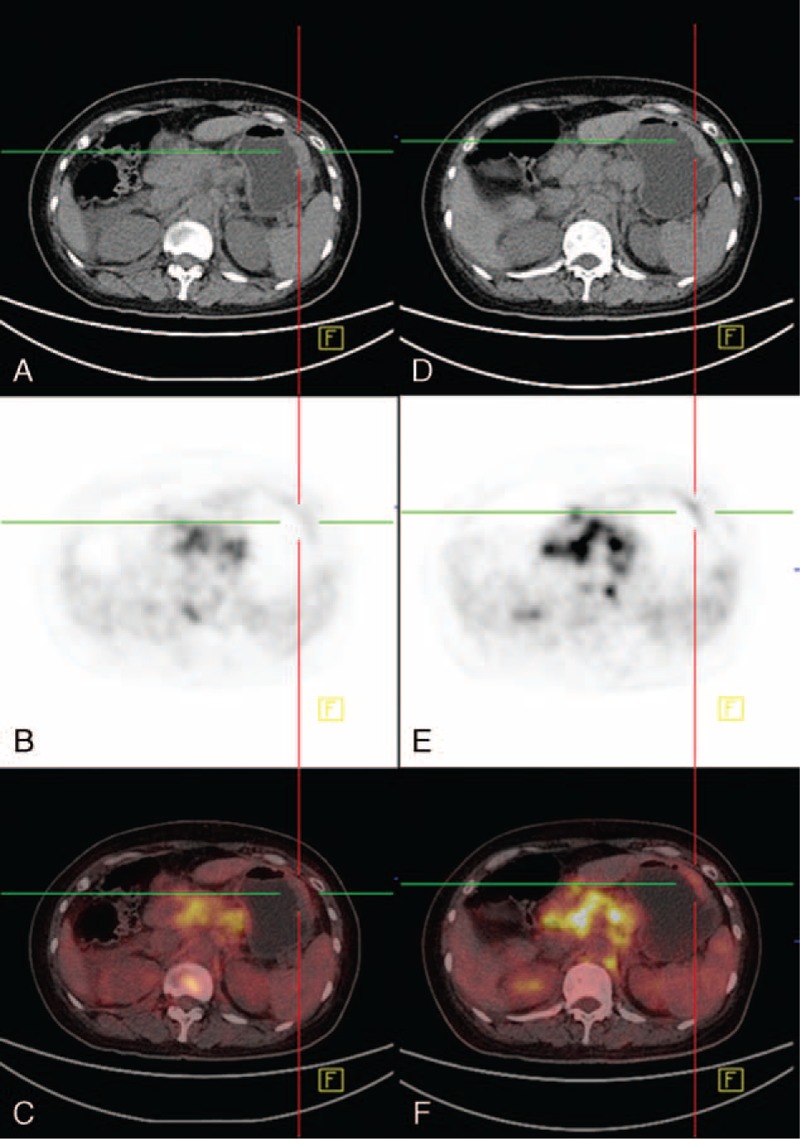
A 51-year-old woman with retroperitoneal lymph node enlargement received PET/CT to find suspicious primary tumors. The early (A–C) and delayed (D–F) imaging were obtained 64 and 142 minutes after tracer injection, SUVmax of diffuse thickening gastric wall was S1 1.9 and S2 3.8, respectively. A poorly differentiated tubular adenocarcinoma of the greater curvature side was confirmed by endoscopy biopsy.
